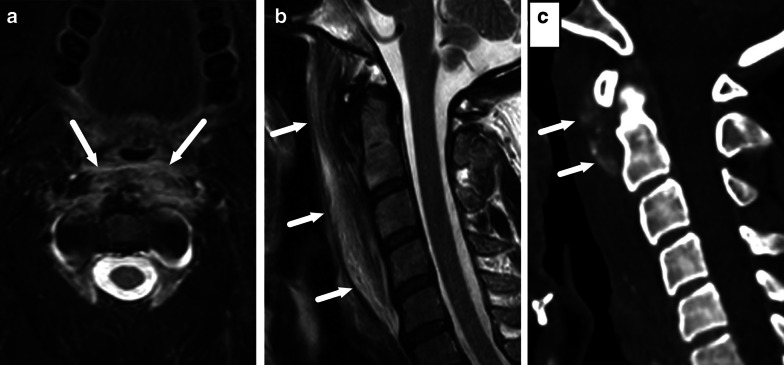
Calcific tendinitis of the longus colli mimicking a retropharyngeal infection. Axial fat-suppressed T2-weighted (a) and sagittal T2-weighted (b) images show massive swelling of the longus colli muscles and retropharyngeal tissues (arrows). A sagittal non-contrast CT (c) shows a pathognomonic finding of amorphous calcifications of the longus colli muscle (arrows)
